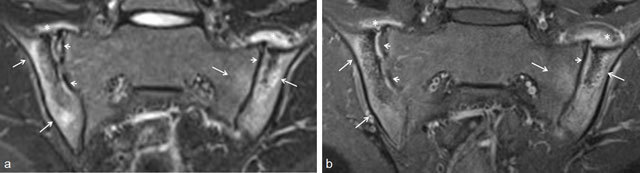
Active and structural sacroiliitis in a 16-year-old girl with JSpA: no added value of Gd. a. Semicoronal STIR image shows high signal in the joint space of both SI joints (small arrows) and bilateral BME (large arrows). Note also the bilateral extensive capsulitis (asterisk). b. Corresponding contrast-enhanced fat-saturated T1-weighted image shows synovial enhancement in both SI joints (short arrows), enhancing osteitis (arrows), and enhancing capsulitis on both sides (asterisk).
